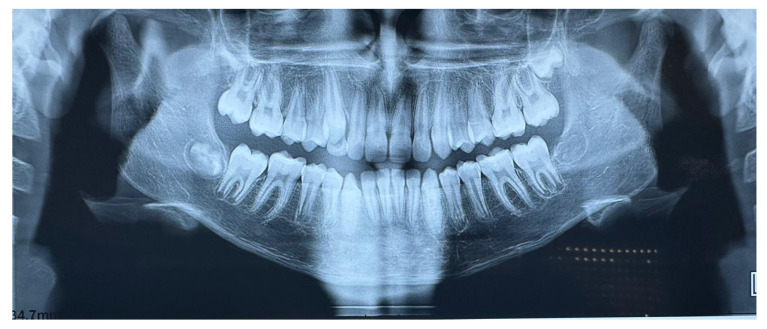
Orthopantomography at T0.
